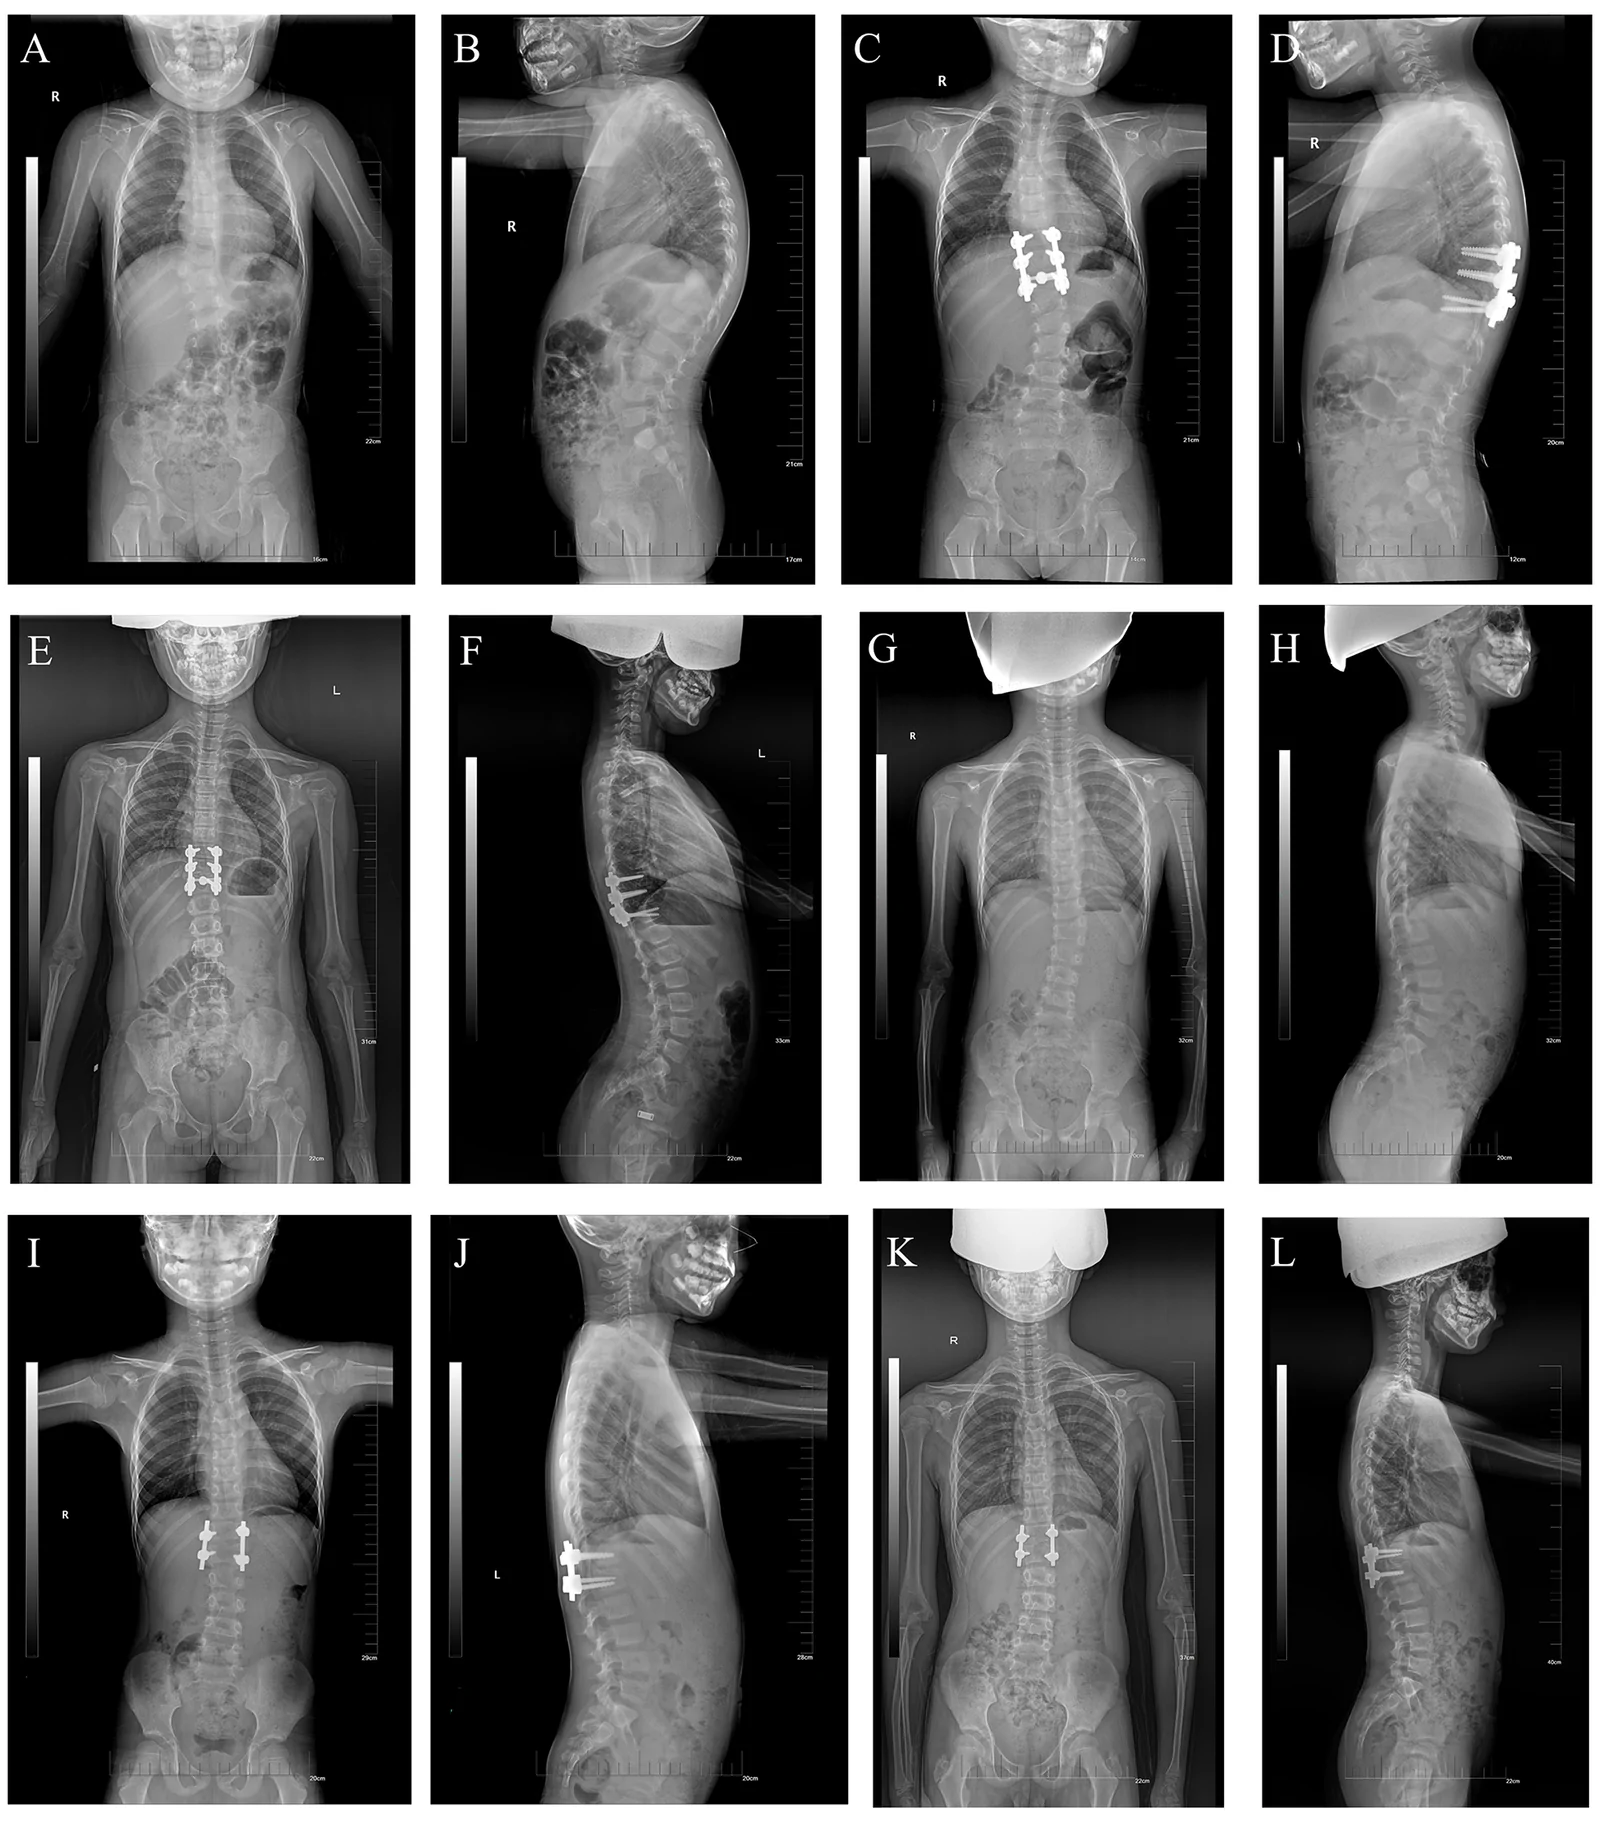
Imaging of different segmental fusion in congenital hemivertebra deformity. All patients were diagnosed with congenital hemivertebra deformity and underwent one-stage posterior hemivertebra resection and pedicle screw fixation in our center. (A, B) Preoperative anteroposterior and lateral radiographs of a 3.17-year-old female patient. (G, H) Preoperative anteroposterior and lateral radiographs of a 6.67-year-old male patient. (C, D, I, J) Radiographs were taken at the follow-up 1 month after the operation, and the flexion was corrected after the application of long- or short-segment fusion. (E, F, K, L) Follow-up images 3 years after surgery, which showed no significant progression of scoliosis after correction.
